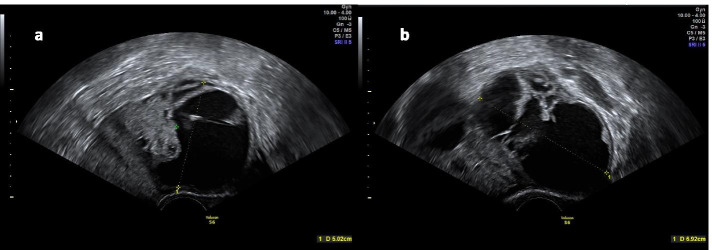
a, b Presentation at vaginal ultrasound. Bilateral adnexal masses with both cystic and solid aspects as well as a remarkable hyperperfusion were noted at vaginal ultrasound. (a) left ovary, (b) right ovary
